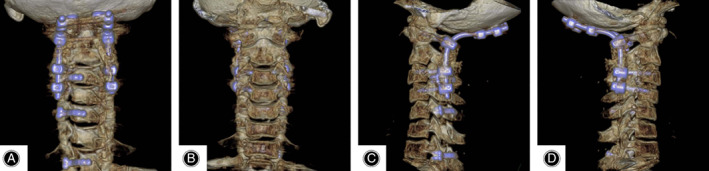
(A, B, C, D) CT reconstruction showed that all the screws penetrated the pedicle into vertebral body accurately from different directions and reconstructed the stability of spinal column. Spinal cord decompression was performed at the compression segment.
